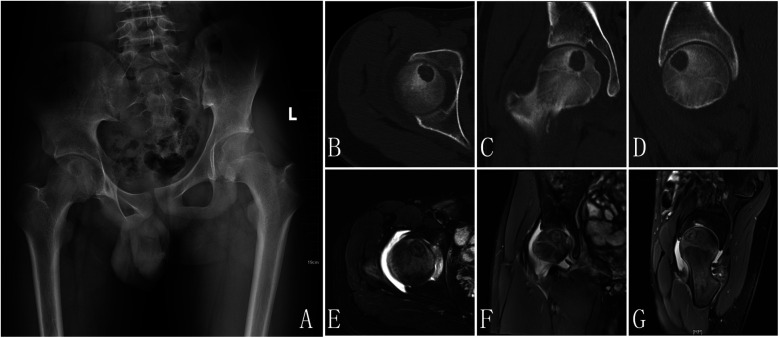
X-ray pre-op: a slightly hypoattenuating shadow was observed in the region of the right femoral head (A), CT: a well-defined, round osteolytic lesion is identified within the right femoral head on coronal imaging. It demonstrates mild hyperdensity with a surrounding sclerotic margin (B–D), MRI: a patchy area of mixed slightly high signal intensity is noted in the right femoral head, with clear margins. Patchy areas of high signal intensity are also present on PDWI in the right femoral head-neck junction and acetabulum. The synovium of the right femoral head is thickened, with associated joint effusion. Additionally, patchy high-signal-intensity regions are observed in the surrounding soft tissues on PDWI (E–G).
